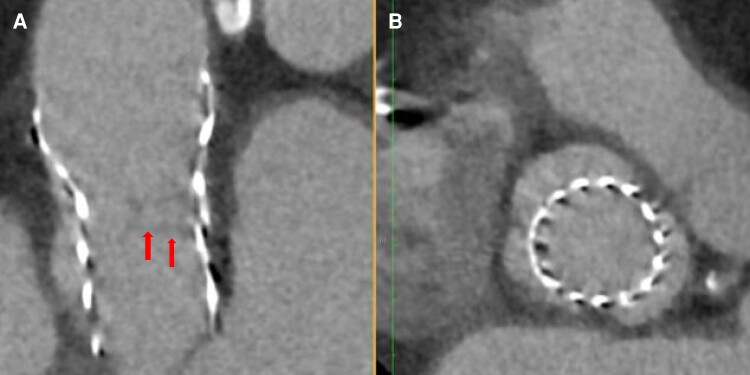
Repeat CT post-TAVR and after 6 months of anticoagulation showing resolution of HALT. (*A*) No evidence of ‘meniscus sign’ seen previously in *Figure 3C*. (*B*) Absence of HALT at the sinuses and leaflets of the aortic valve.
